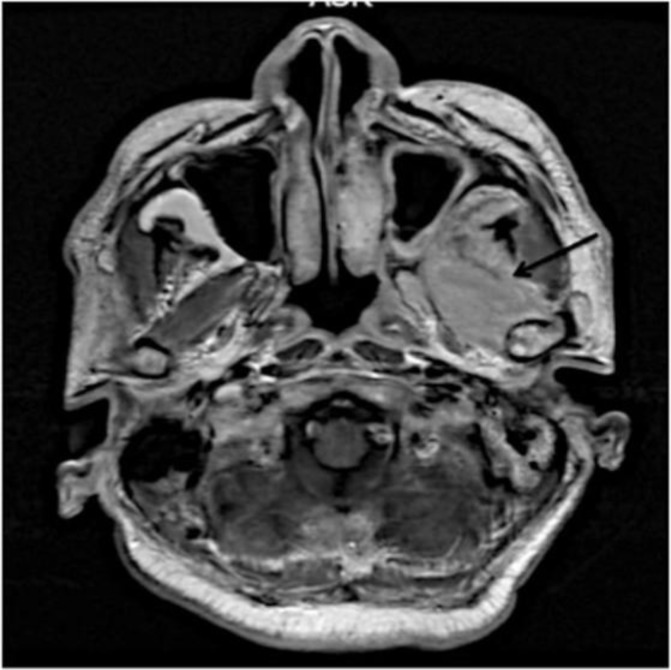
Axial MRI scan demonstrating extensive edema of the left lateral pterygoid muscle in the left infratemporal fossa.
